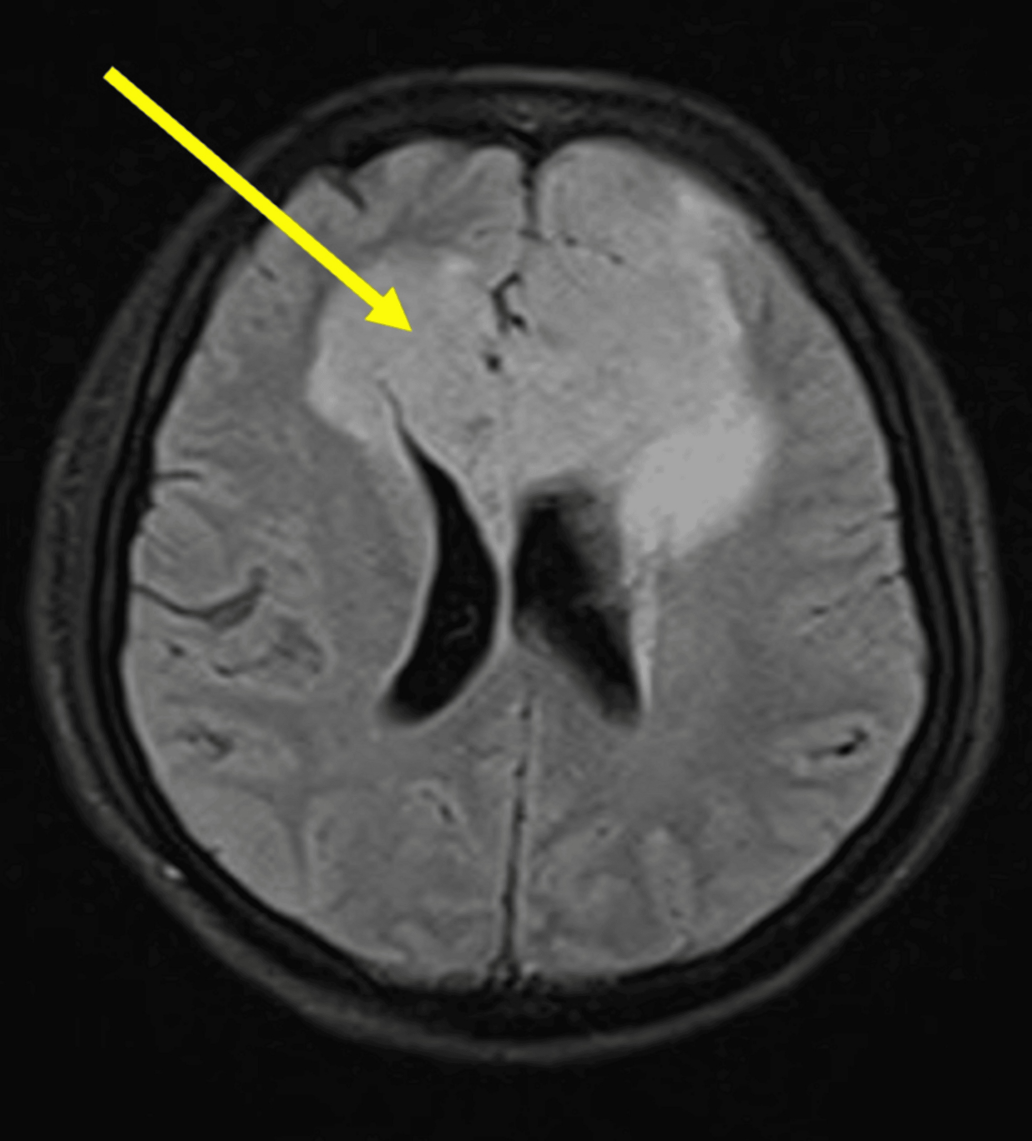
MRI brain showing a large heterogeneous lesion that is hyperintense on T2-weighted and fluid-attenuated inversion recovery (FLAIR) sequences. The lesion is noted in the genu and adjacent body of the corpus callosum, as well as in both frontal lobes and the left basal ganglia.
